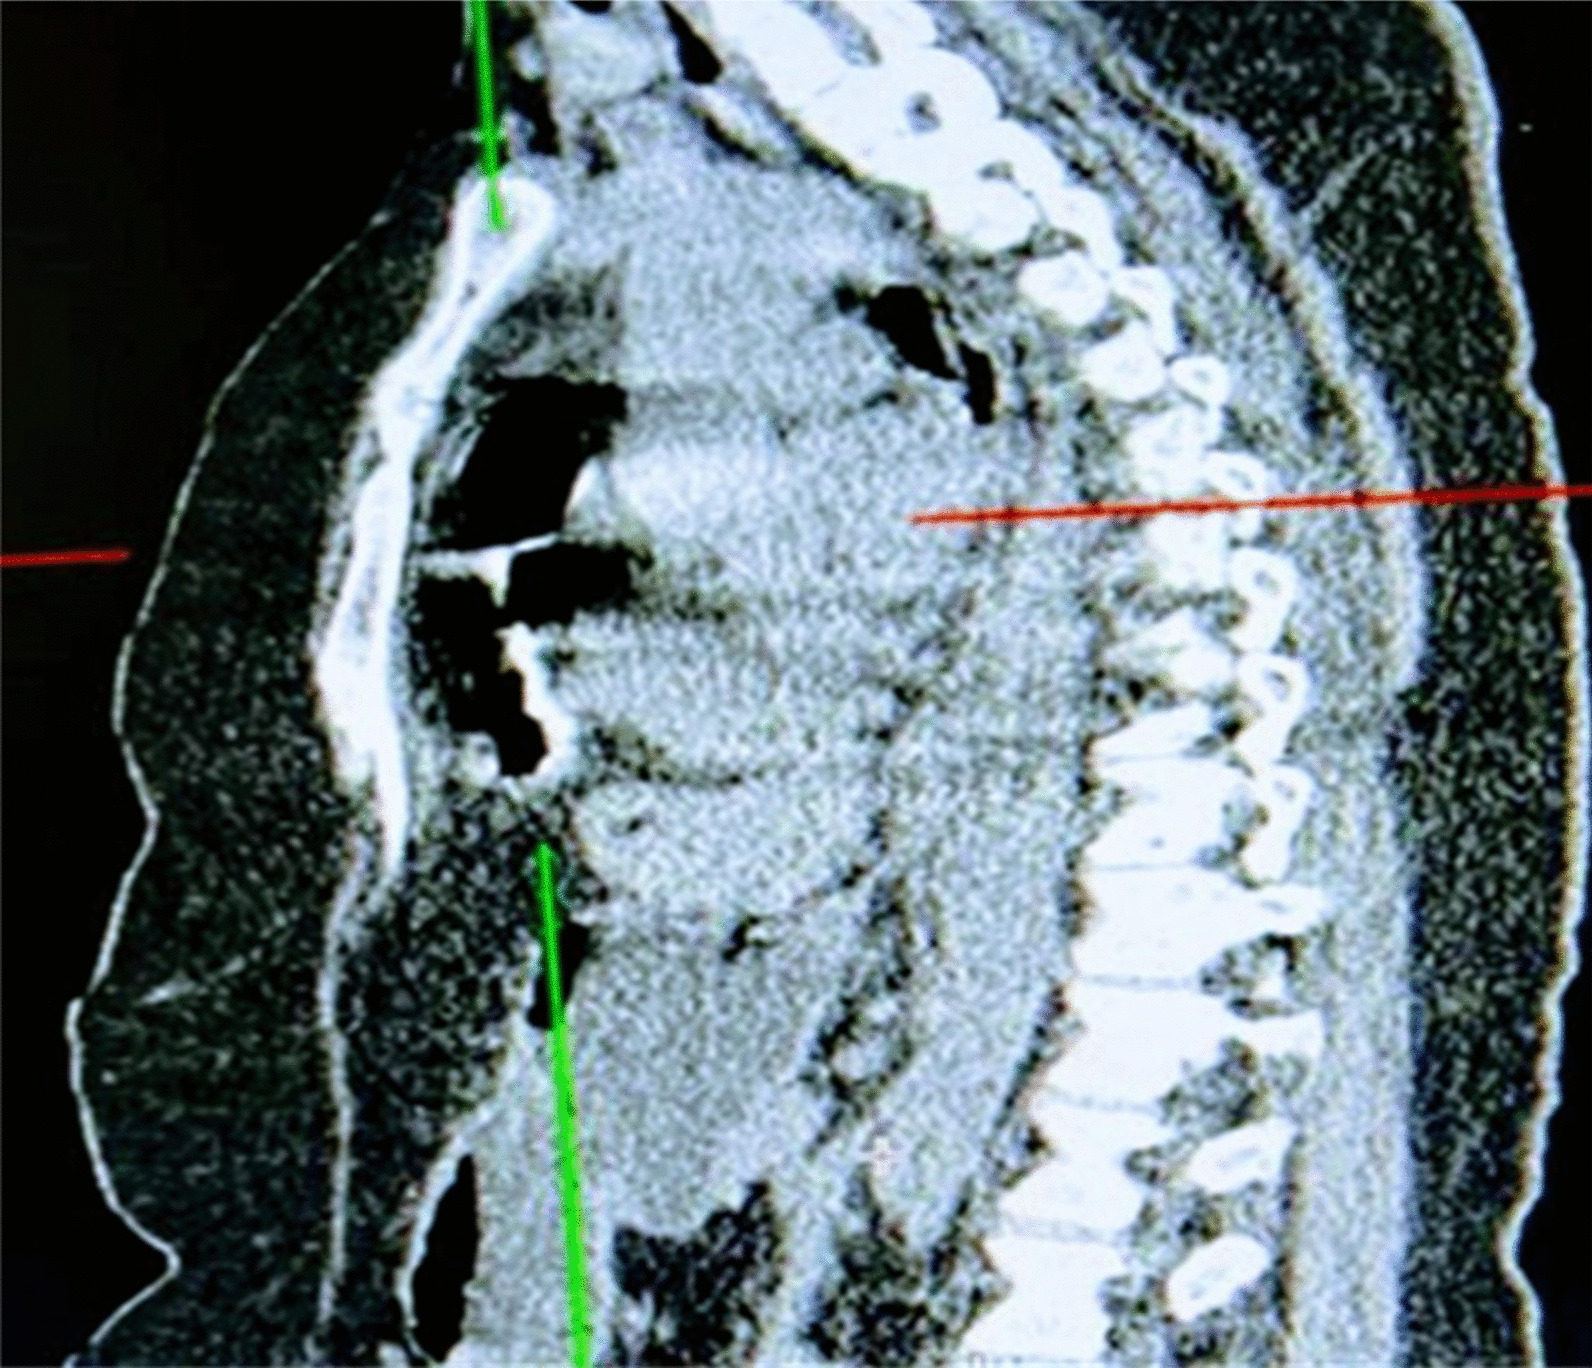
Computed tomography (CT) sagittal view showing a retrosternal mass of fat density and an air-containing viscus consistent with the presence of omentum and transverse colon, respectively
